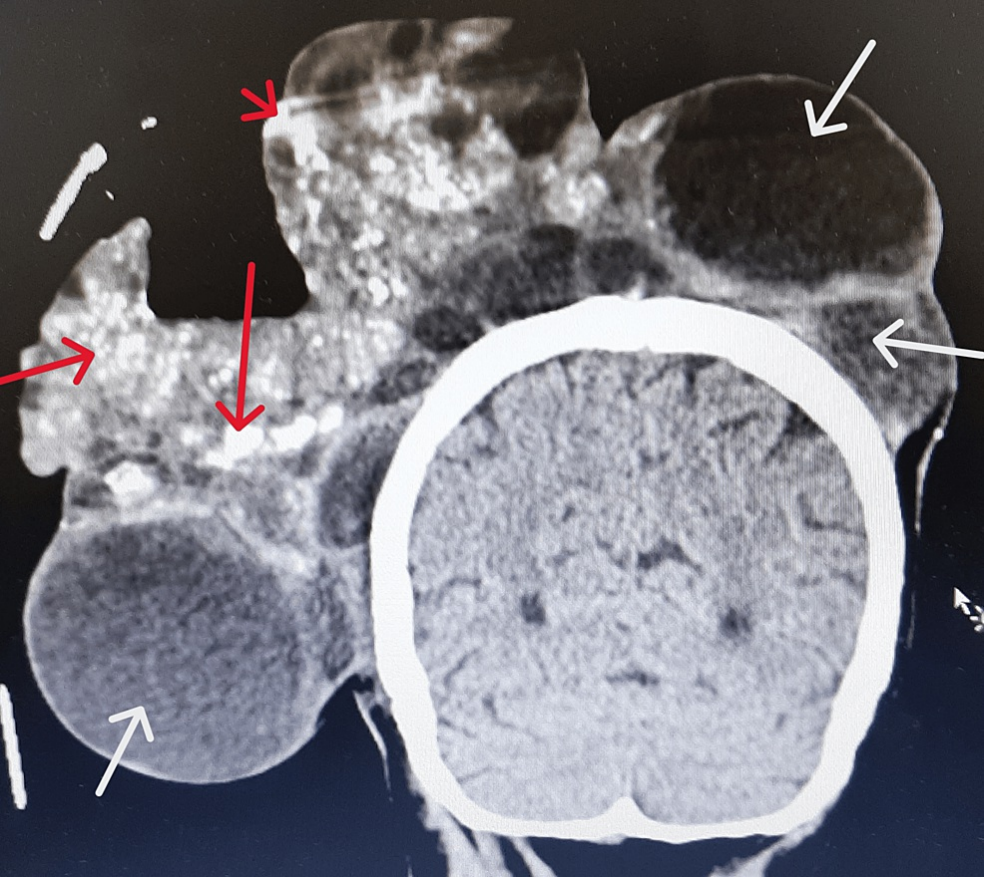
Anteroposterior view of the lesion on CT scan. Some cystic formations (white arrows) can be noted, as well as some calcified hyperdense areas on the lesion (red arrows). CT: computed tomography
